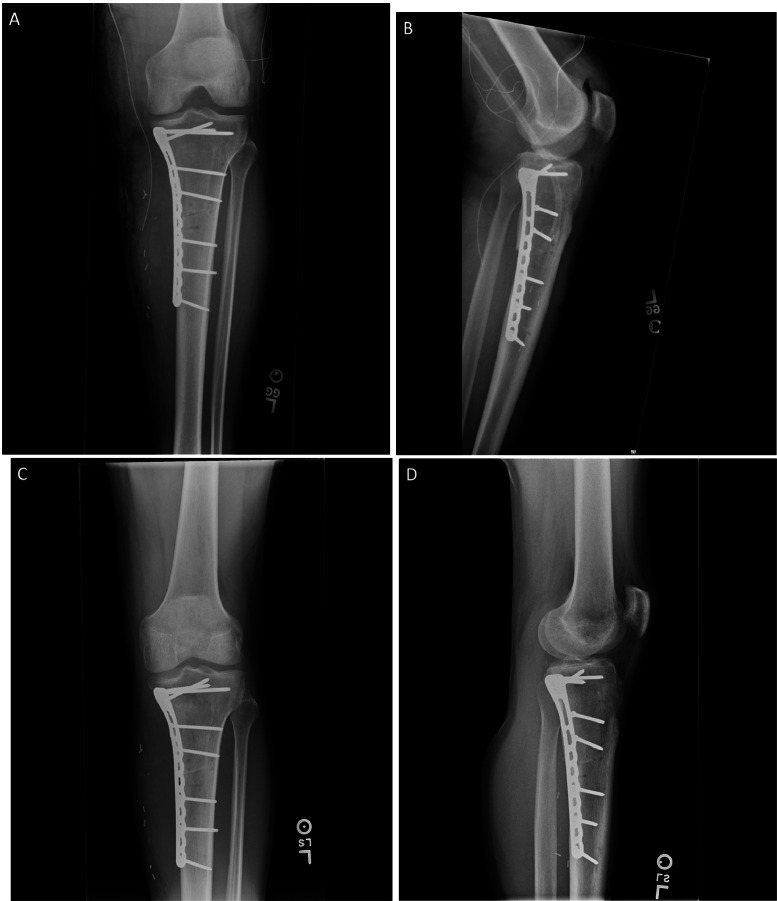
Postoperative radiographs of the left tibia status postsurgical resection of proximal tibial mass with allograft reconstruction. (A and B) Anteroposterior and lateral radiographs obtained 4 days after surgery show a nearly perfect fit of the allograft with medial plate and allograft fixation screws in place. (C and D) Anteroposterior and lateral radiographs obtained 5 months after surgery show progressive incorporation of the allograft.
